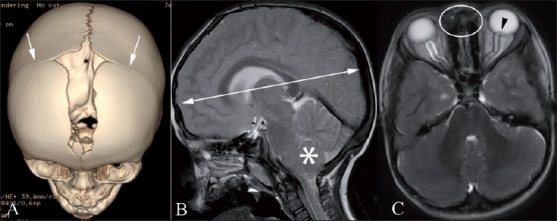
Crouzon’s syndrome. Anterosuperior 3DCT volume rendered image (A), sagittal T2W MRI (B) and axial T2W MRI (C) show premature fusion of the coronal sutures (arrows) and brachycephaly (double-headed arrow). Note also the crowded posterior fossa with onsillar herniation through the foramen magnum (FNx01). There is also hypertelorism (oval). Note the cerebrospinal fluid (CSF)-distended optic nerve sheaths bilaterally and bulging left optic disc (arrowhead) indicating raised intracranial pressure
